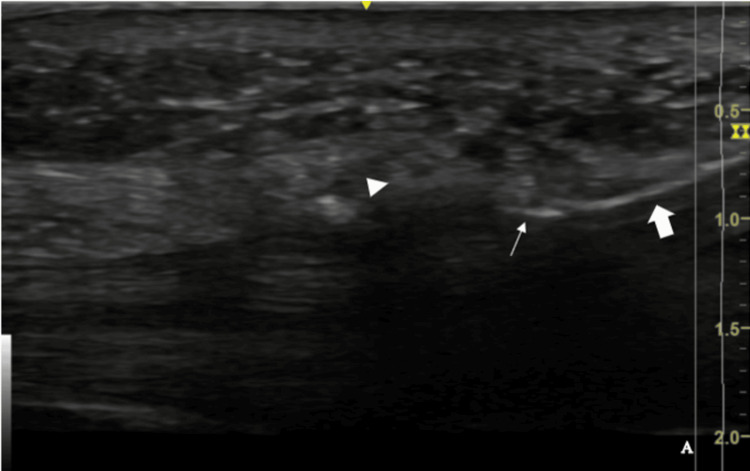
Sonographic long-axis view of the cutting instrument (solid arrow) in contact with the proximal part of the TCL (arrowhead). Note the hyperechoic line at the instrument tip (arrow). This line is generated by wave reflection over the flange located in the blade to show the cutting part of the instrument This figure is the original work of the authors. TCL: Transverse carpal ligament
